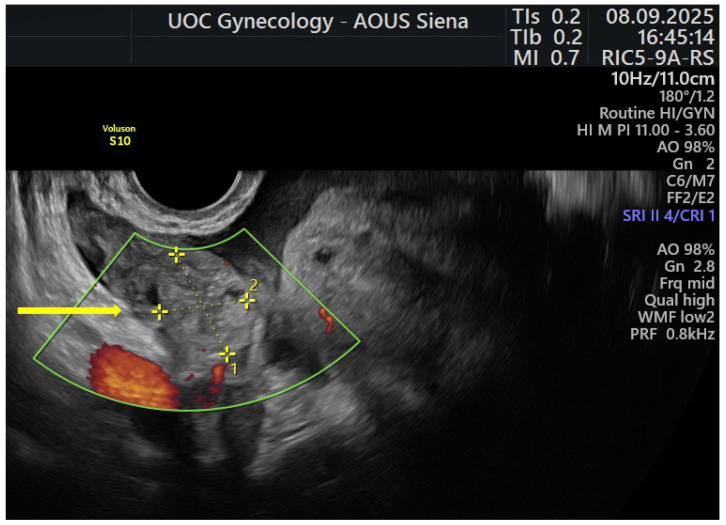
Transvaginal ultrasound showing a right adnexal hypoechoic rounded mass, separate from the ovary (arrow), consistent with the characteristic “blob sign” of early tubal ectopic pregnancy. The yellow dashed calipers (1 and 2) indicate the orthogonal ultrasound measurements used to assess the size of the adnexal mass.
